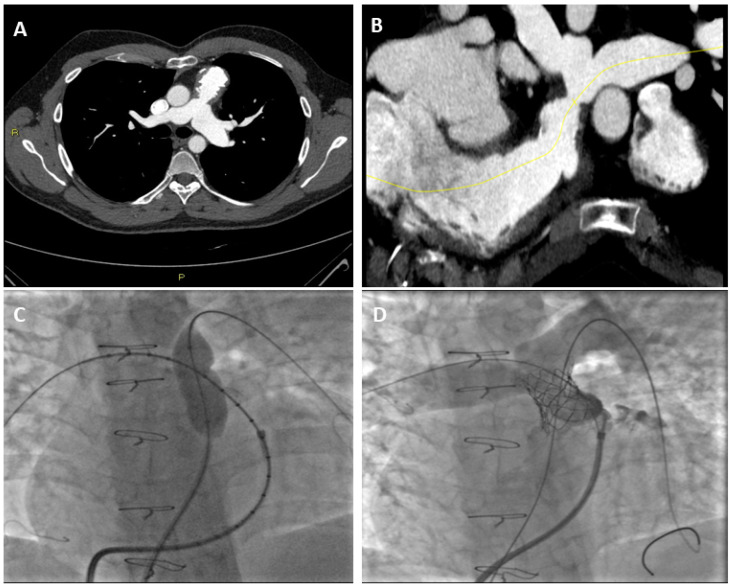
Assessment of Pulmonary Stenosis with CCT in a 15-year-old male patient: (A) Axial CT image with stenosis of the common trunk of the pulmonary artery; (B) CT reconstruction of the vessel using a dedicated post-processing software; (C) Angiographic image of percutaneous transluminal angioplasty (PTA) of the stenotic tract and (D) Angiographic image showing correct stent placement after PTA.
